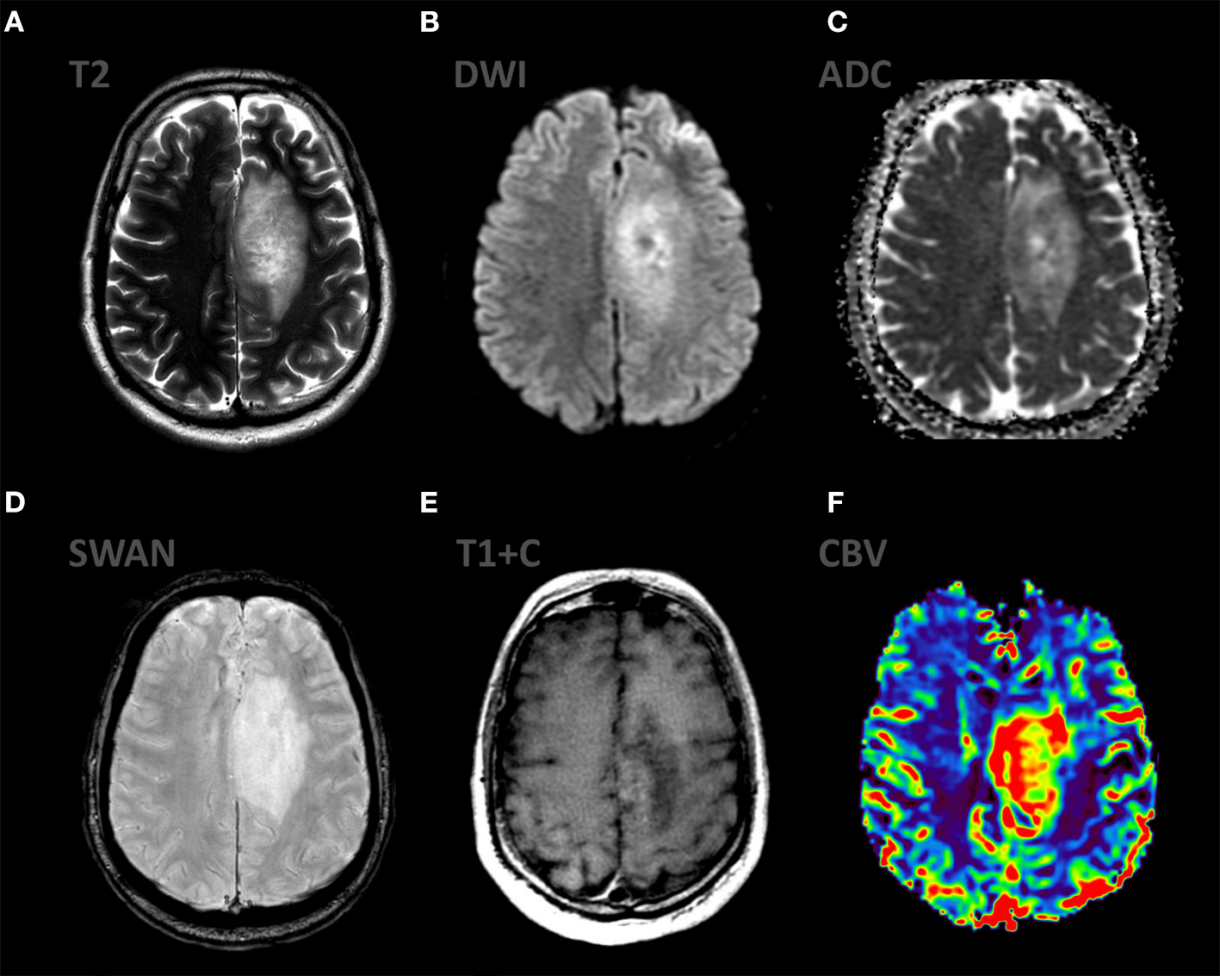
mpMRI ax: (A) T2WI; 6, (B) DWI, (C) ADC, (D) SWAN, (E) T1 + C, (F) DSC-PWI demonstrate the typical appereance of anaplastic astrocytoma (Grade III WHO 2016). There is infiltrative mass in the left frontal region with mild vasogenic edema on T2WI. This mass has low signal on ADC, partial enhancement and high rCBV values.
